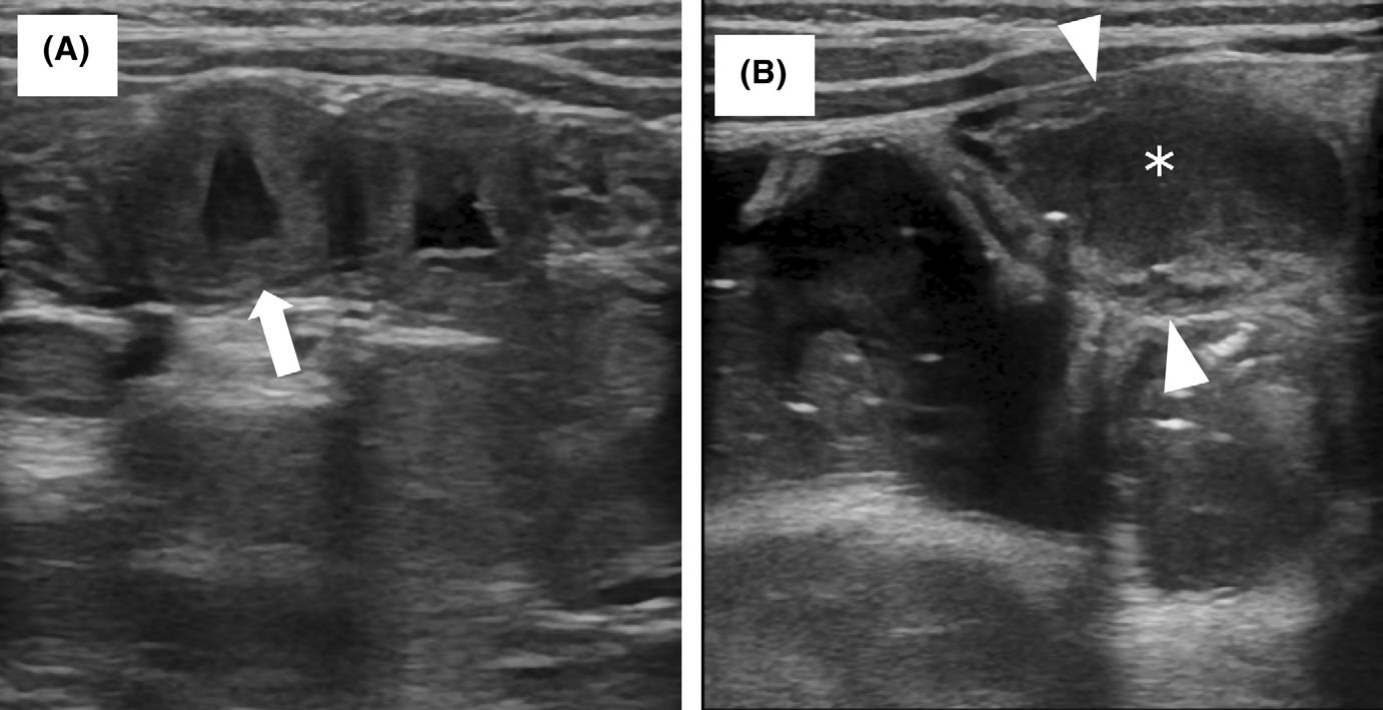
Ultrasonographic findings in the OFC positive group: (A) jejunal wall thickness with edema (arrow), measurement by placing one pointer at the mucosal layer and the other at the serosa in the same loop; (B) fluid accumulation depicted as a hypoechoic lesion (*) with bowel dilatation in the ileum (arrowhead). OFC, oral food challenge.
